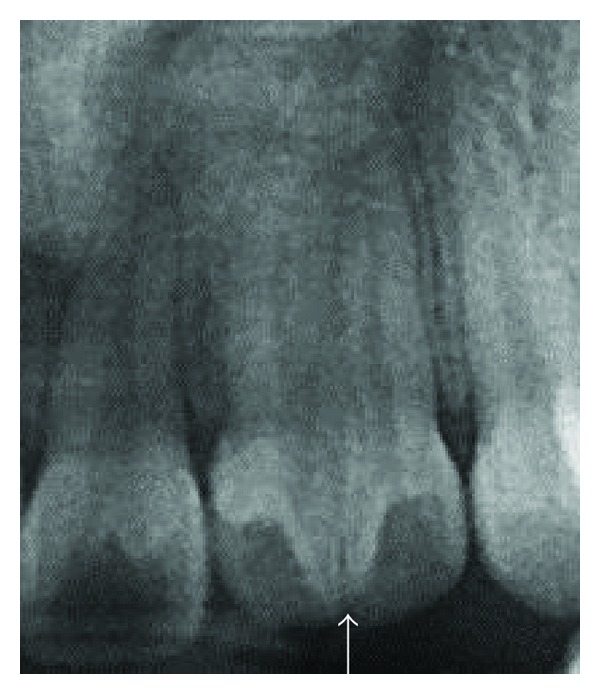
Preoperative radiograph revealing midroot connections between the root canals.
